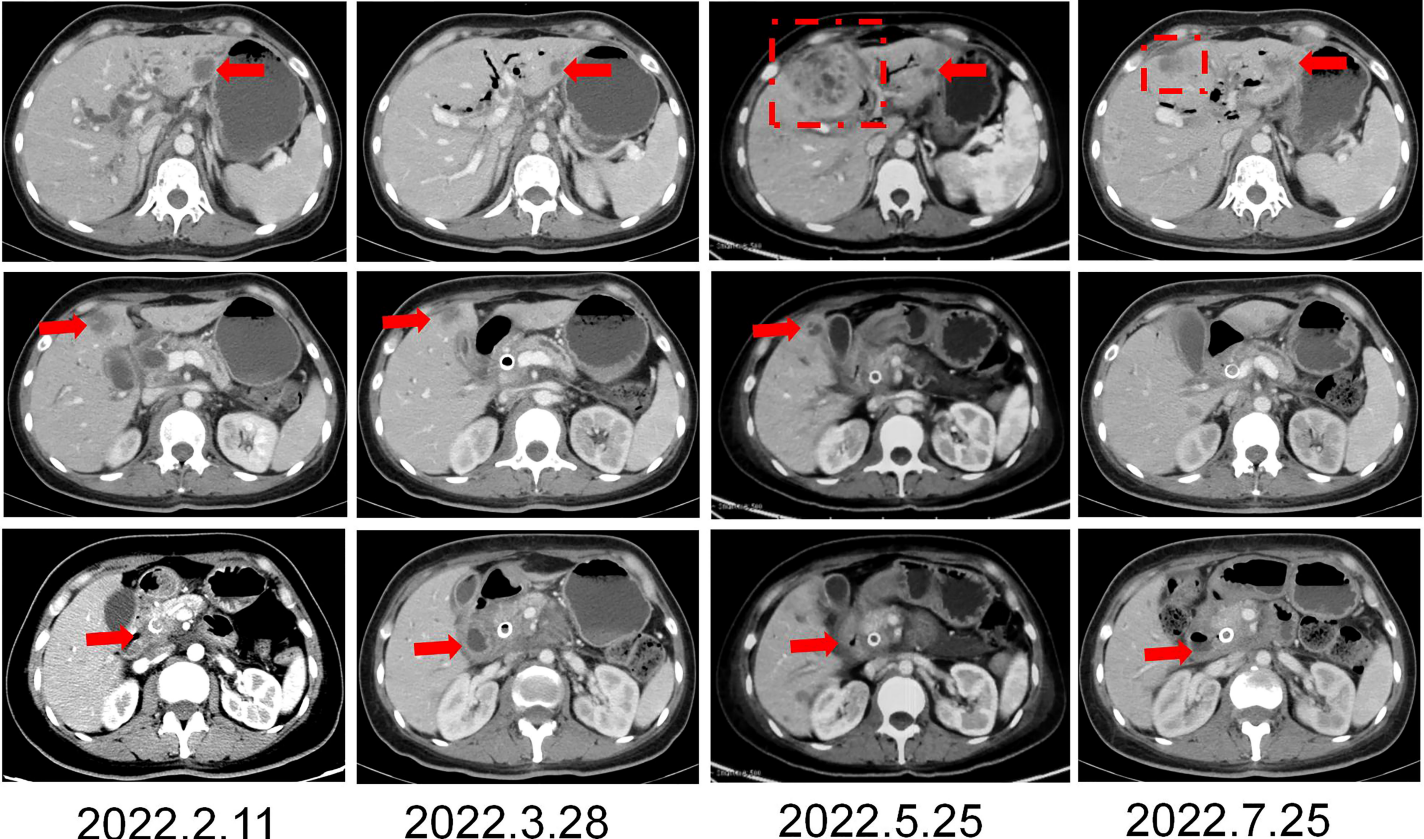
CT imaging changes in the course of furomertinib treatment.*
